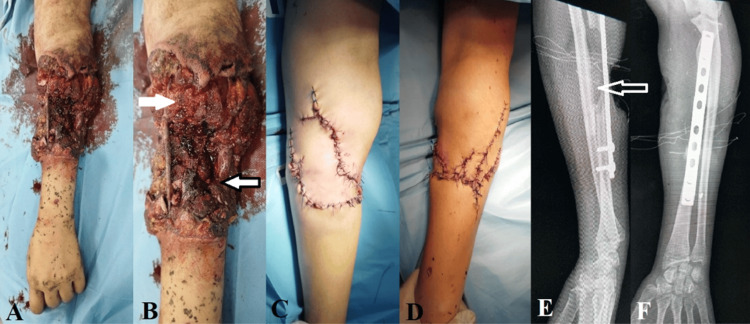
Initial injury and primary surgical management (A, B) Initial injury photographs demonstrating the severity of the injury, with extensive soft-tissue destruction and severe contamination. (C, D) Intraoperative photographs from the primary surgery showing soft-tissue debridement and initial wound closure with radius fixation using a plate. (E, F) Postoperative anteroposterior (F) and lateral (E) radiographs demonstrating the radial bone defect.
